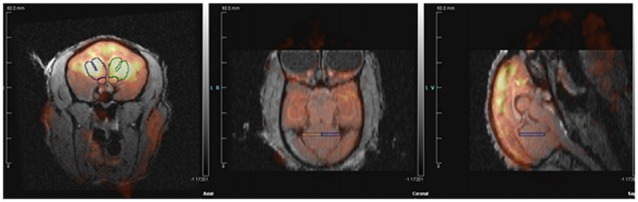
Positron emission tomography (PET) image co-registered with structural magnetic resonance imaging (MRI).
